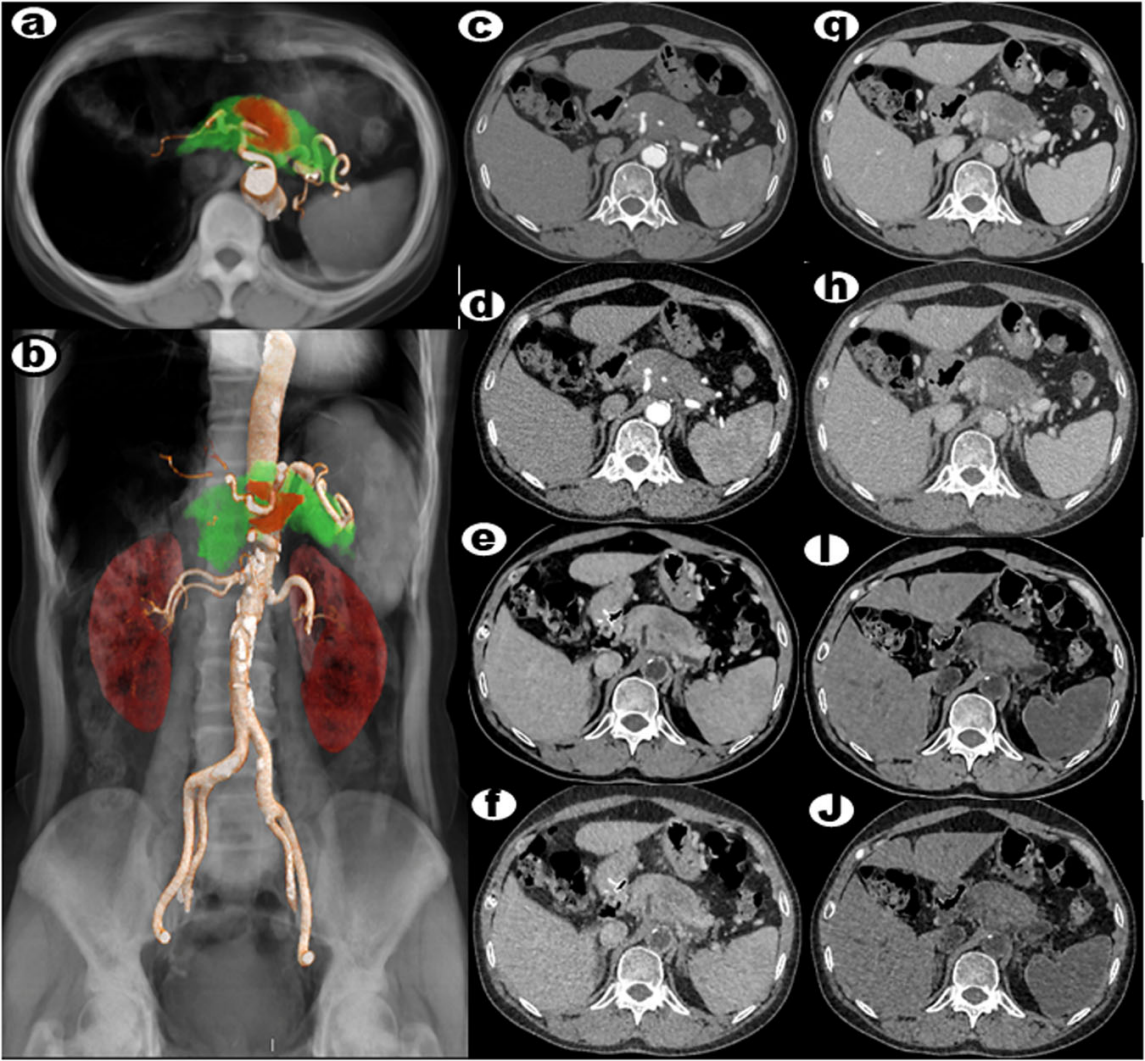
A 56-year-old woman presented with PDAC located in the body region of the pancreas. a, b Global illumination rendering (GIR) fusion images reconstructed using the AiCE algorithm were used to visualize the tumor invasion. In these fusion images, normal pancreatic parenchyma was displayed in green, while the tumor was depicted in red. c–f Axial CT images at the arterial phase were reconstructed using AiCE, AIDR 3D, AiCE–BBCT, and AIDR 3D–BBCT algorithms, revealing the presence of a pancreatic tumor (indicated by an arrow). g–j Similarly, axial CT images at the portal venous phase, reconstructed using the same algorithms, confirmed the presence of the tumor (also indicated by an arrow). The image reconstruction utilized a slice thickness of 1 mm. These imaging findings provide a visual representation of the PDAC and demonstrate the application of various algorithms, including AiCE, AIDR 3D, AiCE–BBCT, and AIDR 3D–BBCT, in detecting and assessing the tumor at different phases of contrast enhancement
